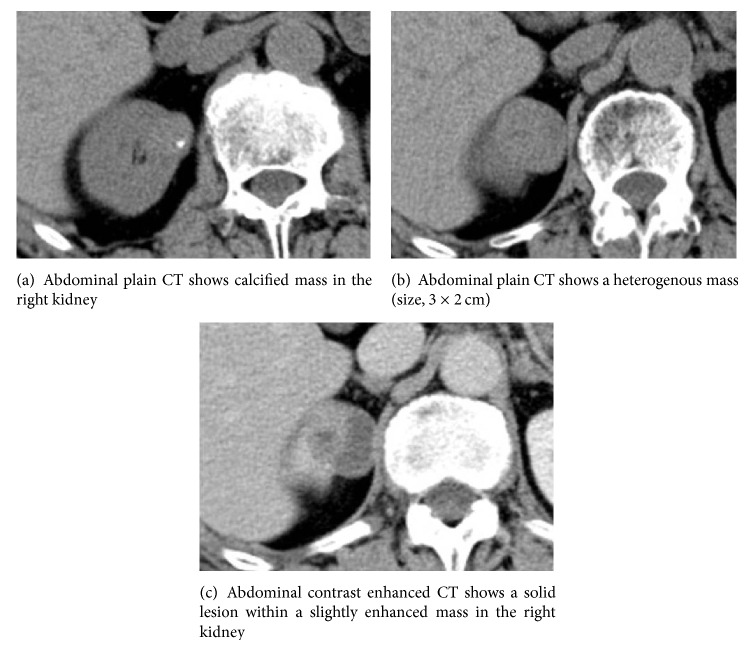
Abdominal computed tomography (CT).
